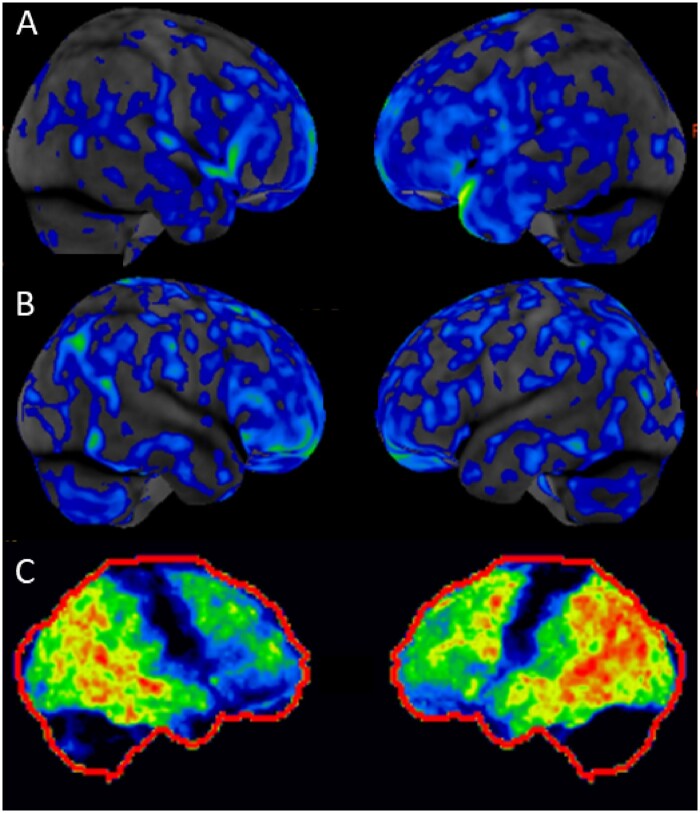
Representative FDG-PET scan findings in two patients diagnosed with autoimmune encephalopathy and one patient with probable neurodegenerative disorder. FDG-PET images, lateral views. (A) Diffuse hypometabolism (patient 32, Table 2). (B) Frontal and temporal hypometabolism (patient 17, Table 2). (C) Profound temporal and parietal hypometabolism and mild frontal hypometabolism (patient 45, Table 6).
